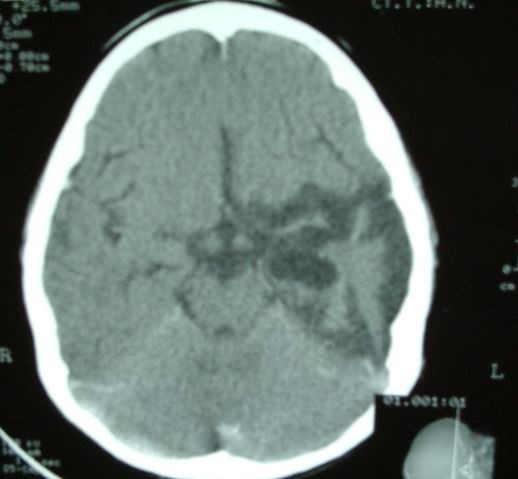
A, B: Follow-up CT scan demonstrates severe brain atrophy in previous hypodense area which confirms infracted brain was resolved.
